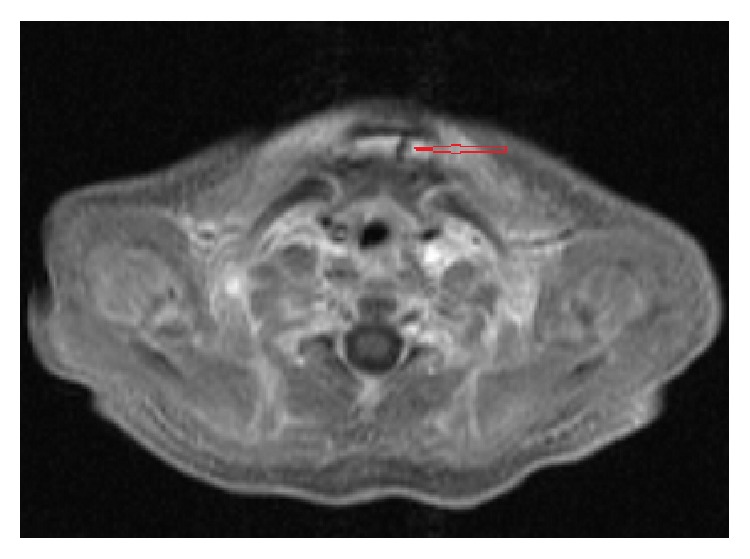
Postgadolinium fat saturated T1 axial MR image of the neck shows midline low T1 signal blind ending cleft in the skin/subcutaneous fat (red open arrow).
